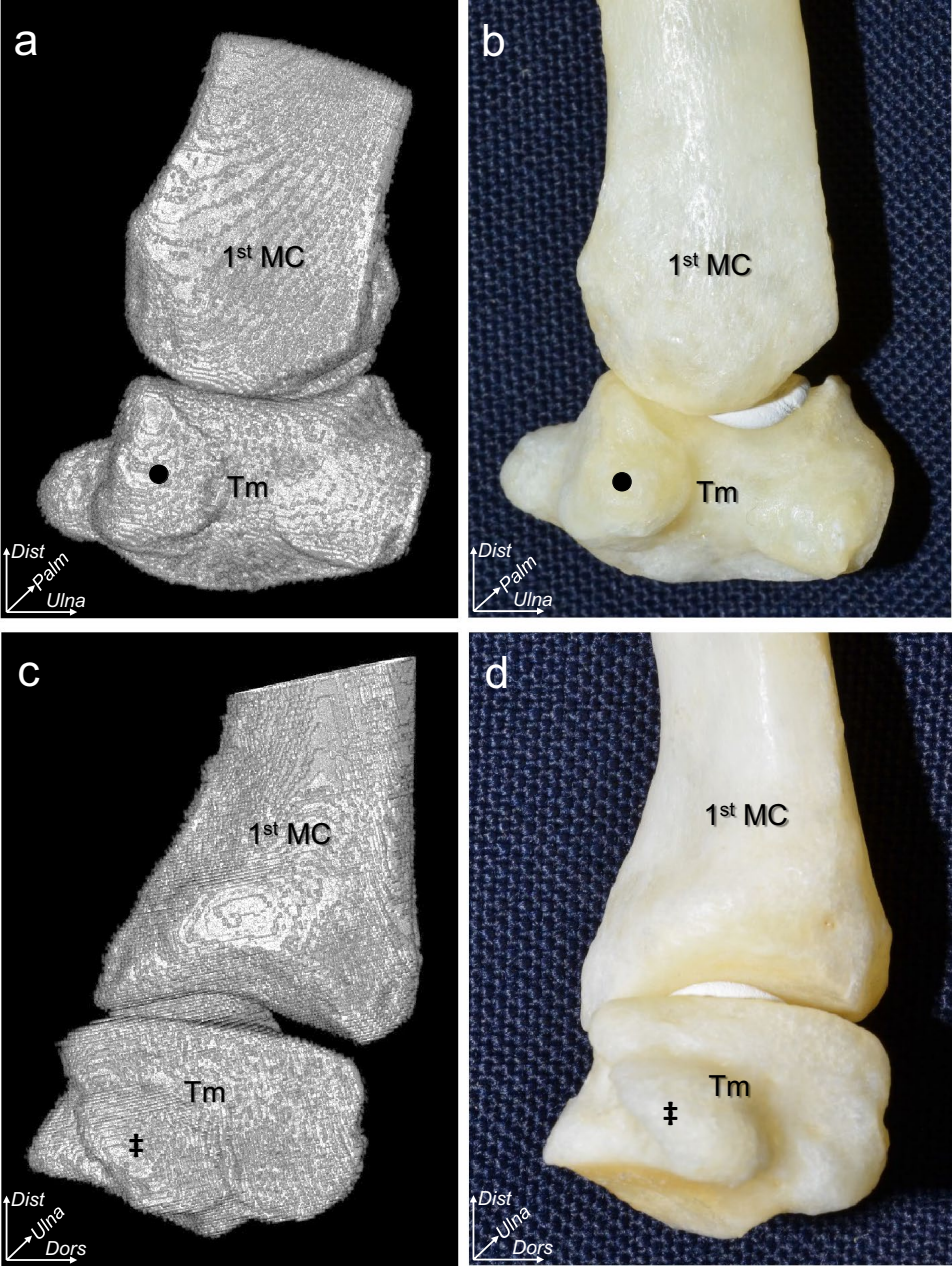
Bone morphology of the trapeziometacarpal joint. Three-dimensional (3D) computed tomography images and actual bone images of the dorsal (a and b) and radial aspects (c and d) of the trapezium (Tm) and the first metacarpal bones of the right thumb. (a) the Tm is prominent (black circle) on the radial side of the dorsal aspect. (b) the actual bone images of (a). (c,d) 3D images and actual bone images of the radial aspect of the trapeziometacarpal joint, respectively. The double-dagger shows the radial roof of the flexor carpi radialis tendon groove. *Dist*, distal; *Ulna*, ulnar; *Dors*, dorsal; *Palm*, palmar.
